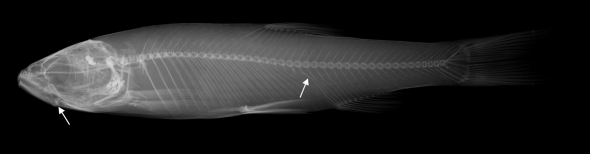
Radiograph of Squalius janae, PZC 406, 133.2 mm SL, Croatia: Boljunscica, showing 24+20 total vertebrae (arrow shows first caudal vertebra) and lower jaw length (arrow shows posterior end of lower jaw).
